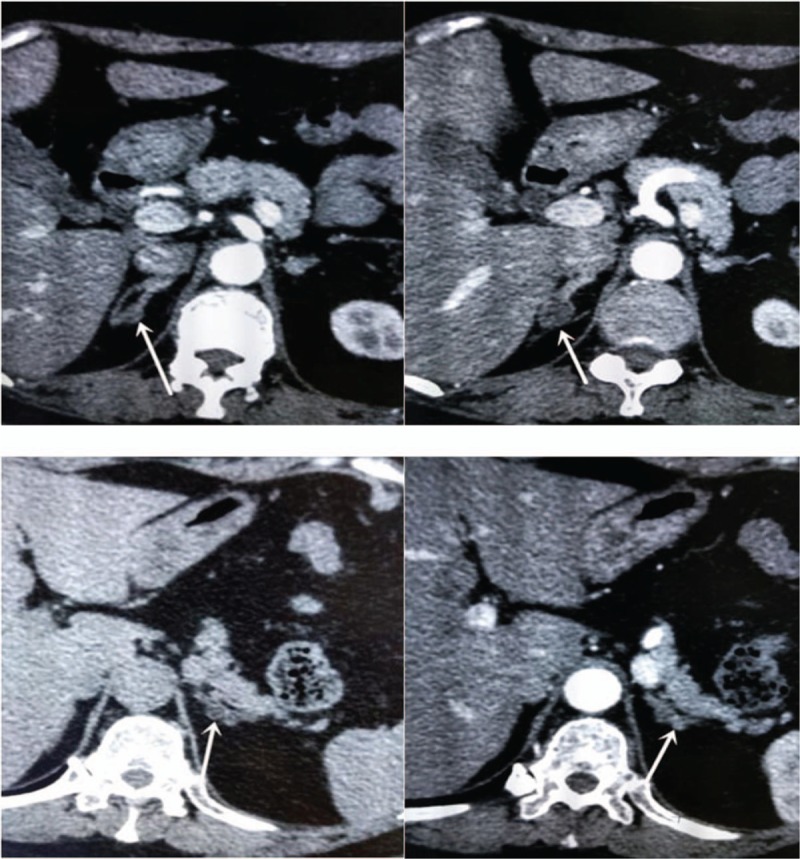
Bilateral adrenal enhancement computed tomography (CT) scan. The arrows indicate the bilateral adenomas.
